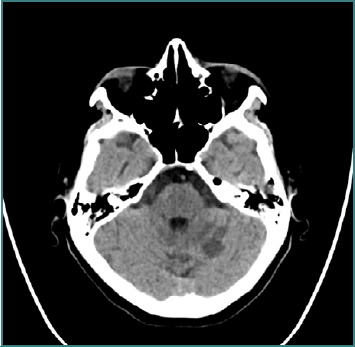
Non-contrast brain CT performed 24 hours after acute treatment, demonstrating acute ischemia in the left anterior cerebellar lobe and left anterior cerebellar peduncle, without evidence of intracranial hemorrhage
